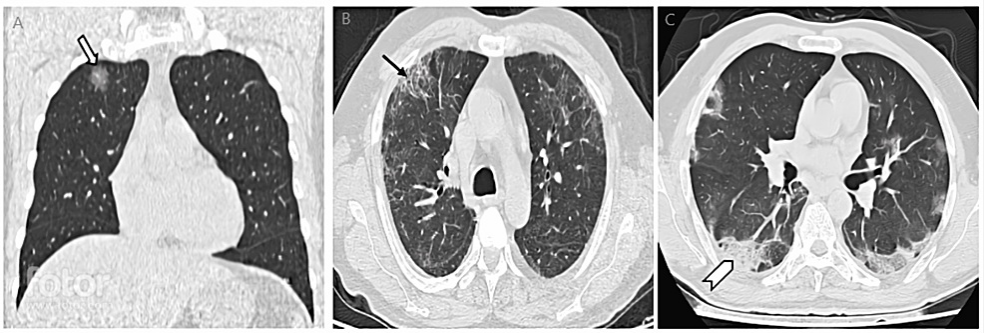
HRCT images of patients classified in mild category. A: Coronal image showing focal GGO in right upper lobe (mild category). Block arrow showing focal ground glass opacity. B: Axial image showing GGOs with adjacent fibrotic changes (mild category). Arrow showing peripheral fibrotic changes. C: Axial image showing GGOs with surrounding consolidatory changes (mild category). Chevron shows peripheral involvement in form of a consolidatory patch. Note focal involvement of lung parenchyma in the mild category. GGO, ground glass opacity; HRCT, high resolution computed tomography
